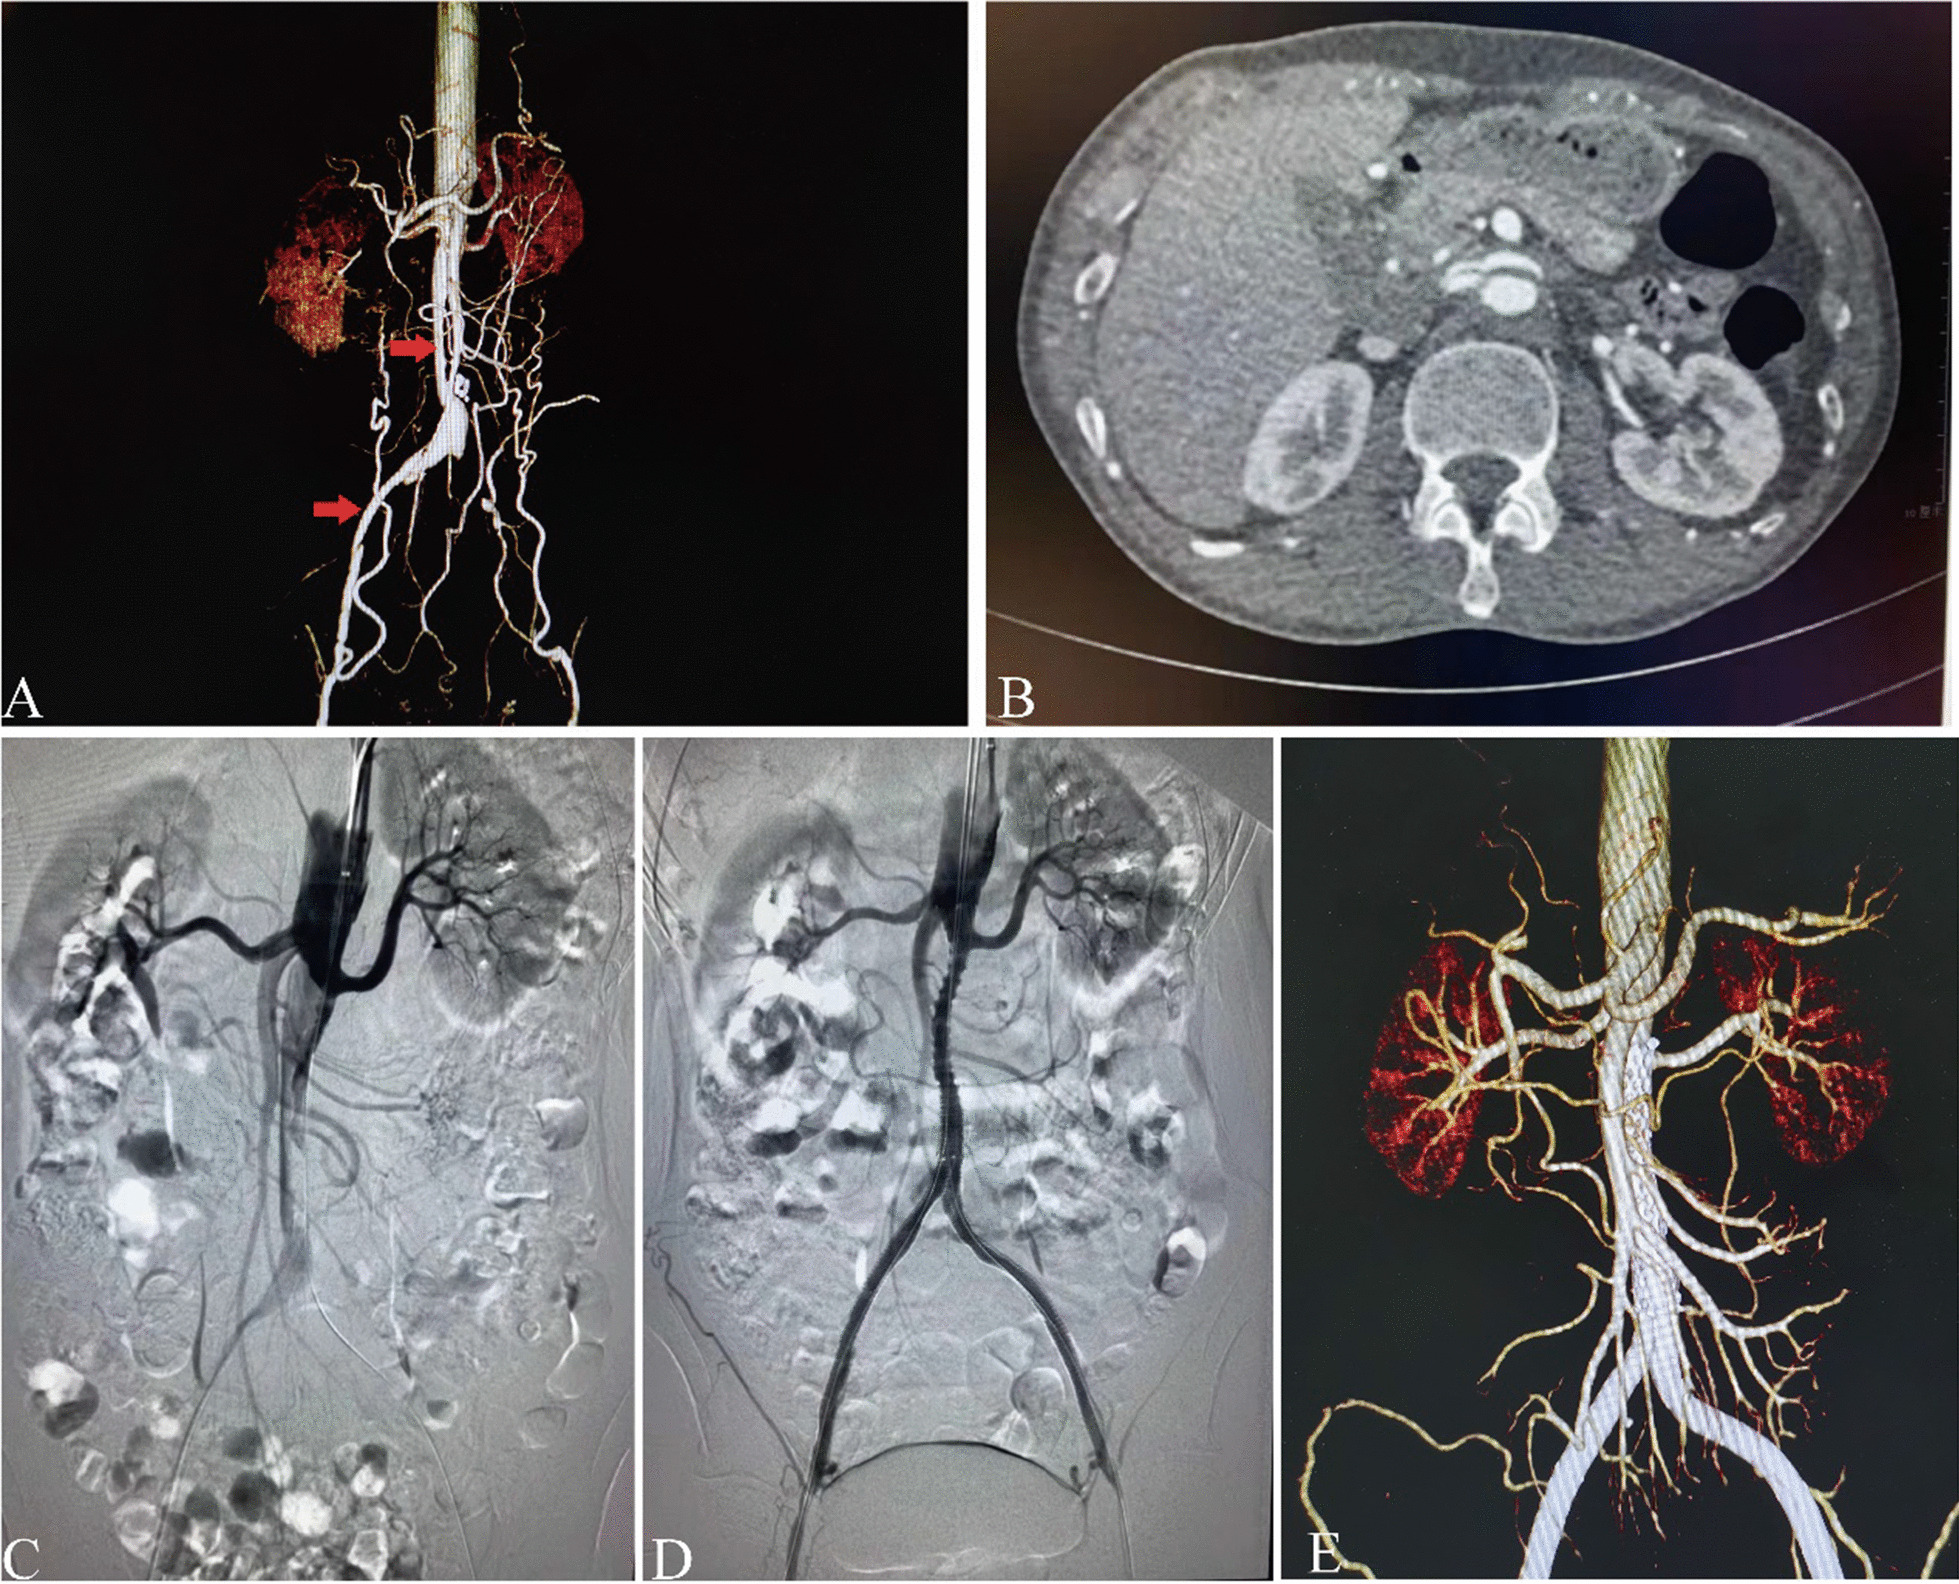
A 57-year-old male patient presented to a local hospital with intermittent claudication. After examination at the local hospital, the patient was diagnosed with AIOD with TASC II D lesion and received endovascular treatment at the local hospital. However, due to the limited technical level of the local hospital, the operation was not successful, so the patient was urgently transferred to our center. Preoperative CTA indicated the dissection of the patient's right external iliac artery abdominal aorta (A, shown by the red arrow), and right renal artery (B). The patient was transferred to our department for emergency angiography and stent implantation, and the operation was very successful (C, D). The stent position and patency were well demonstrated by reexamination 3 months after the operation (E)
